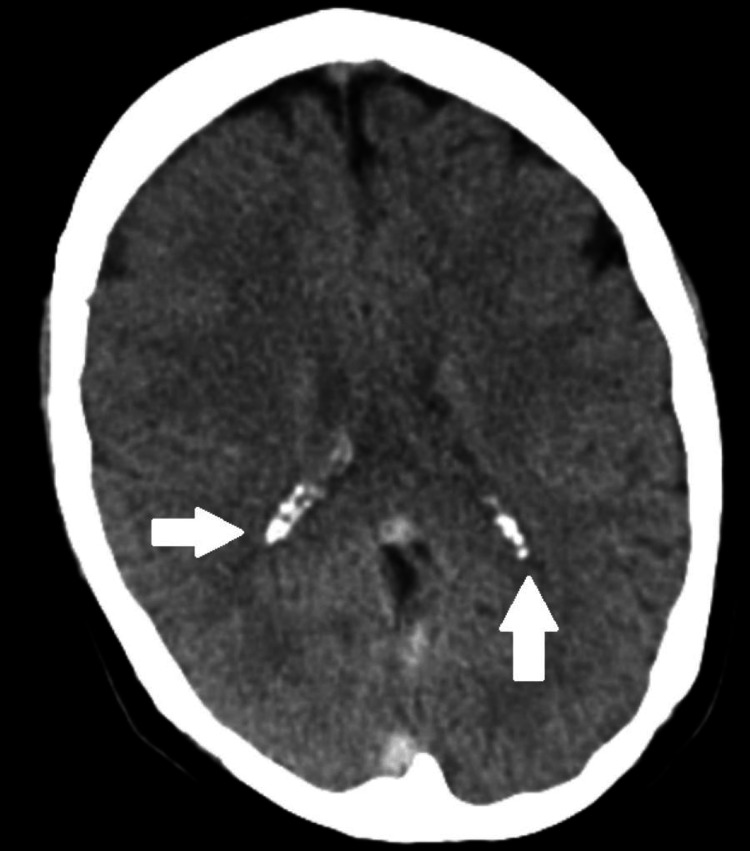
Computed tomography scan of the brain showing venous thrombosis
